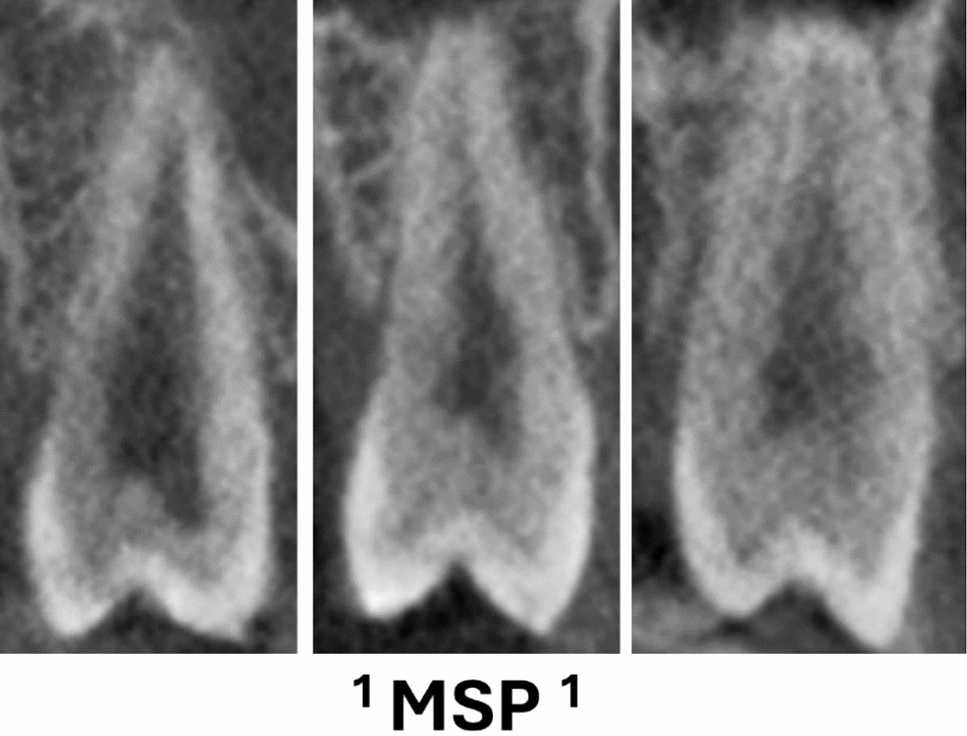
CBCT images (sagittal view) of maxillary second premolar (MSP) showing single code root canal morphology
